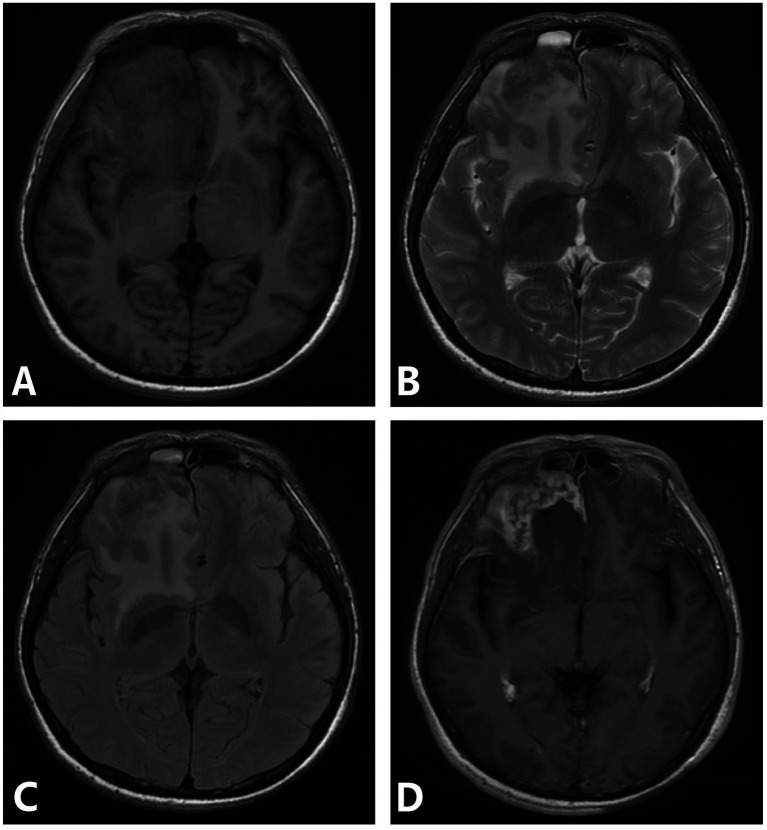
(A–D) Syphilis gumma type neurosyphilis, a 39-year-old male presented with intermittent suspiciousness and behavioral abnormalities for 4 days. MRI revealed an irregular lesion in the right frontal region with a surrounding edematous zone, showing heterogeneous signal intensity and marked heterogeneous enhancement with adjacent meningeal involvement. Panel (A) (T1WI): demonstrates an irregular isointense lesion in the right frontal lobe; panel (B) (T2WI): shows hyperintense signal within the lesion and surrounding edema; Panel (C) (FLAIR): depicts extensive hyperintense signals highlighting perilesional edema; Panel (D) (T1WI-CE): reveals marked heterogeneous enhancement with adjacent meningeal enhancement.
